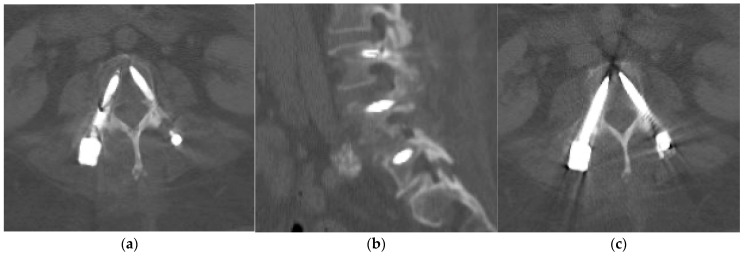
Axial (a) and sagittal (b) CT bone reconstructions with MAR showing a linear dark band across the mid-section of the right L3 pedicle screw. Axial CT bone reconstruction without MAR (c) at the same L3 level showing intact screws.
